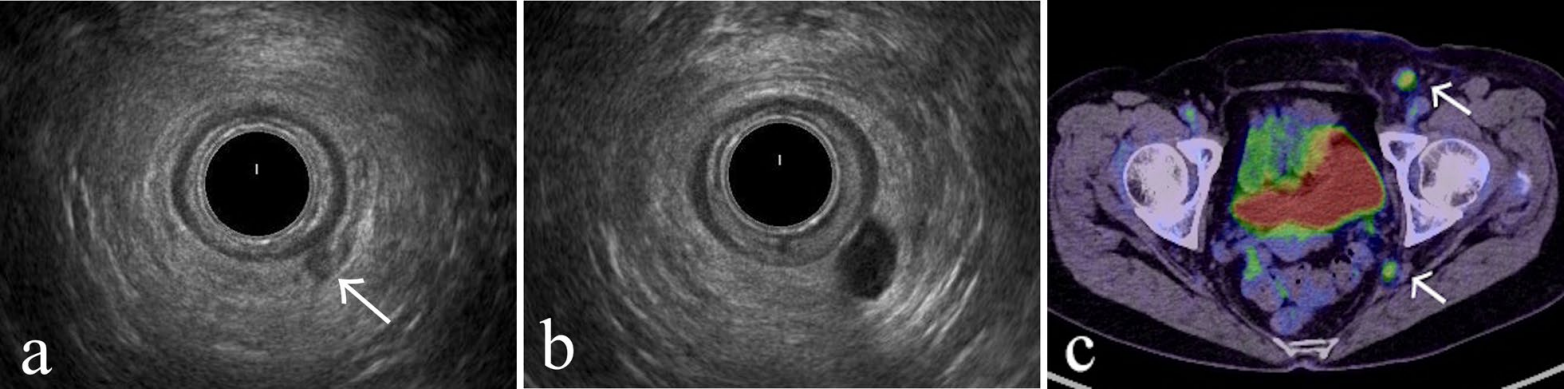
Imaging studies. a Transanal ultrasonography revealed a fistula-like structure with unclear boundaries in the intersphincteric layer at the time of the perianal abscess 3 years previously (arrow). b Anal fistula was expanded with hypoechoic content at the time FAMC was diagnosed. c [18F]-Fluoro-2-deoxy-d-glucose (FDG)–positron emission tomography/computed tomography showed lymphadenopathy in the right internal iliac and inguinal regions with abnormal FDG accumulation (arrows)
